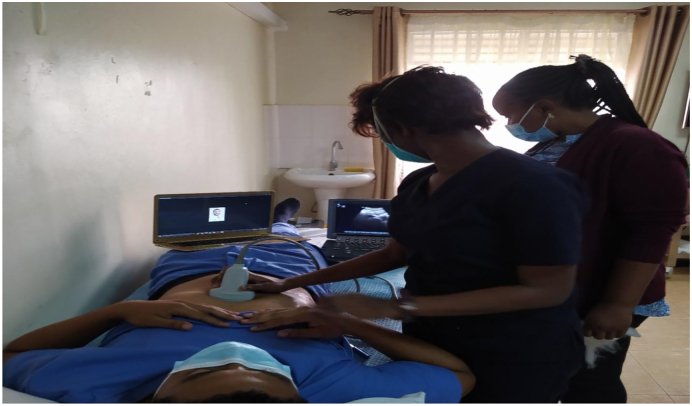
Arrangement for virtual instruction. Both the trainee and the ultrasound screen are visualised by the virtual trainer being performed on a volunteer.6.Participant flow: It is easier and more feasible to have participants rotate through the stations than trainers. This allows each station to be tailored to each topic, thereby appropriating materials much more efficiently, including ultrasound machines. See Fig. 2 below
